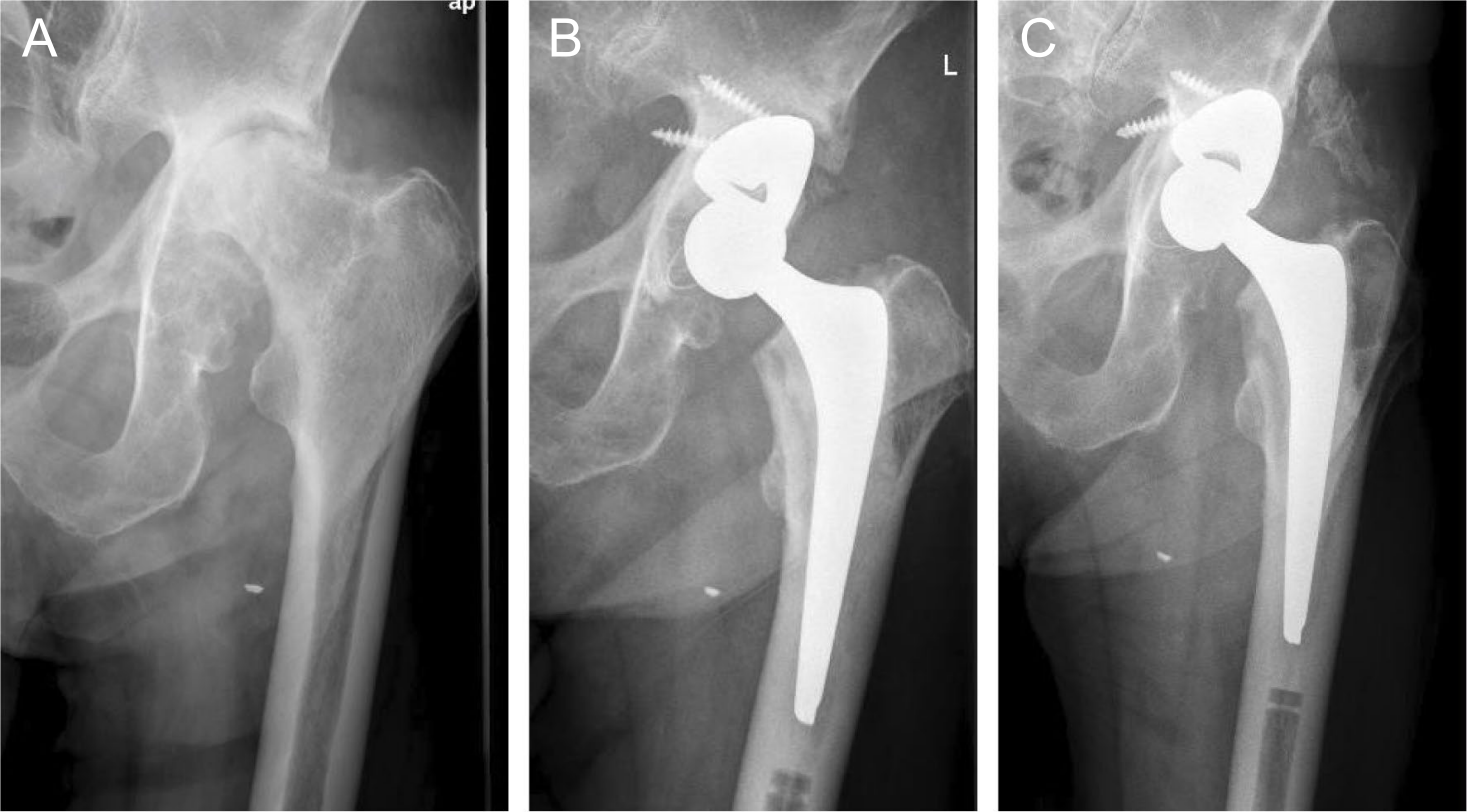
A Preoperative anteroposterior radiograph of the hip of a 91-year-old patient with severe osteoarthritis with advanced acetabular bone loss. B Anteroposterior radiograph of the same patient 3 month postoperatively, with a tantalum TM augment in combination with a cemented polyethylene cup. C Anteroposterior radiograph of the same patient 3.1 years postoperatively, with signs of loosening and dislocation of the tantalum augment with broken screw with unchanged cup position
